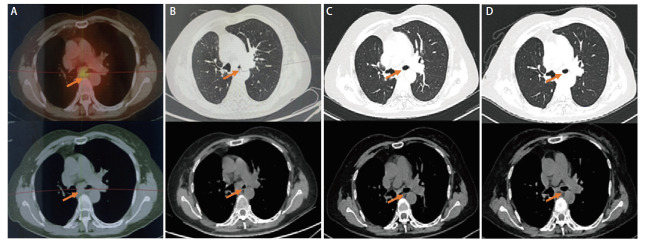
小细胞肺癌影像学表现。A：2023-08-28 PET/CT：左主支气管略狭窄；B：2023-10-08胸部CT：肺窗（上）、纵隔窗（下）可见左主支气管较2023-08-28 PET/CT狭窄程度明显加重；C：2024-05-13即服用阿来替尼7个月后复查胸CT：肺窗（上）、纵隔窗（下），可见左主支气管狭窄明显改善；D：2024-10-23即服用阿来替尼12个月后复查胸部CT：肺窗（上）、纵隔窗（下），未见疾病进展。
